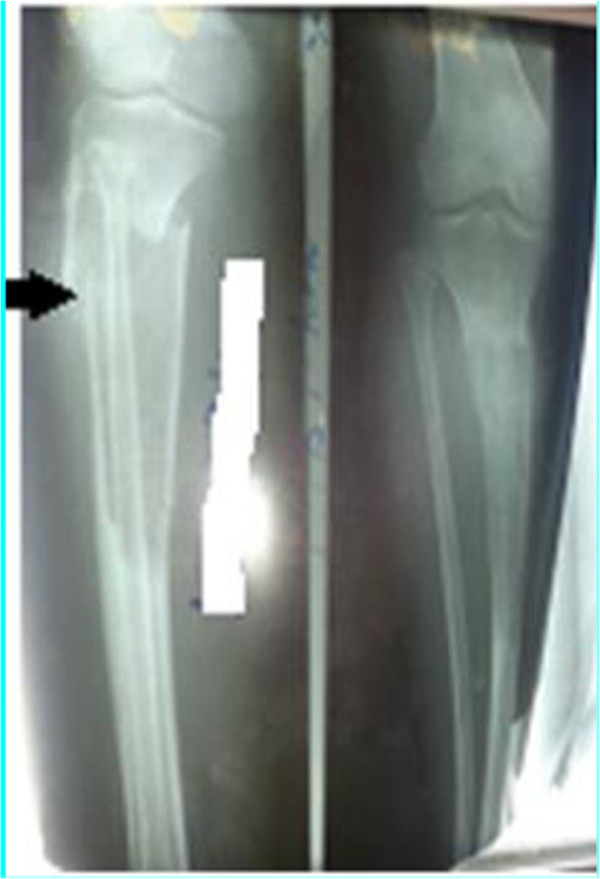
Preoperative X-ray of the right leg (arrow).
